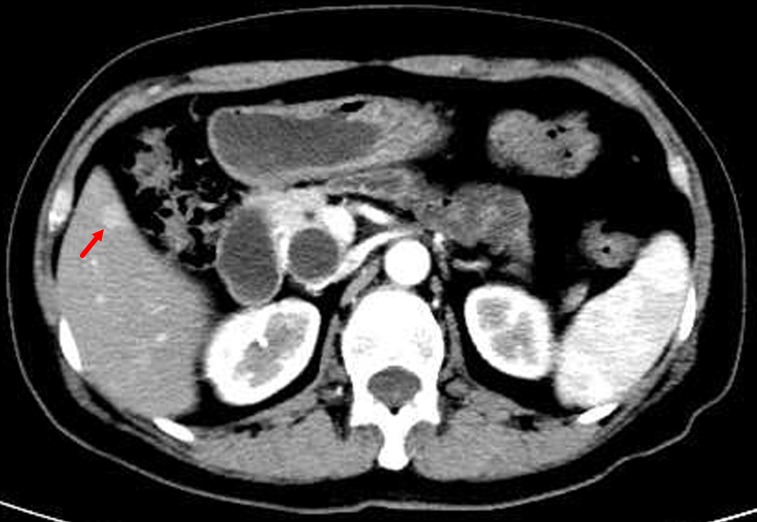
TACE in a 59-year-old female with multiple liver metastases form pancreatic neuroendocrine tumor A. Contrast-enhanced CT before TACE revealed a segment 6 hypovascular liver metastasis in the arterial phase (arrow) B. contrast-enhanced CT showed a significant decrease in the lesion at 6 months after treatment (arrow).
